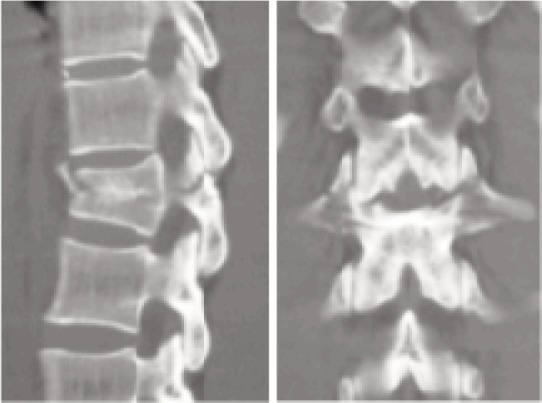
Pre-operative CT sagittal and coronal views of L1 fracture (AO Type B 2.3).
